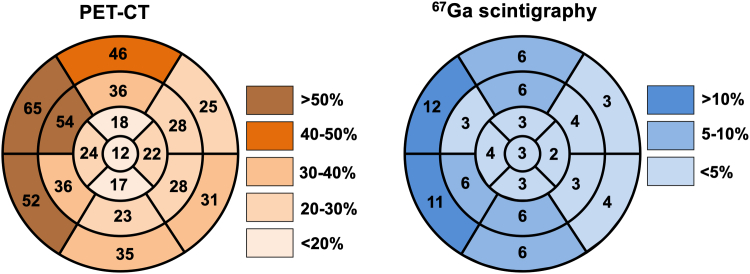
The Difference in Positivity Rates Between FDG-PET and ^67^Ga Scintigraphy FDG-PET was positive in 97.0% of patients, whereas ^67^Ga scintigraphy was positive in only 25.7%. CT = computed tomography; other abbreviations as in Figure 1.
